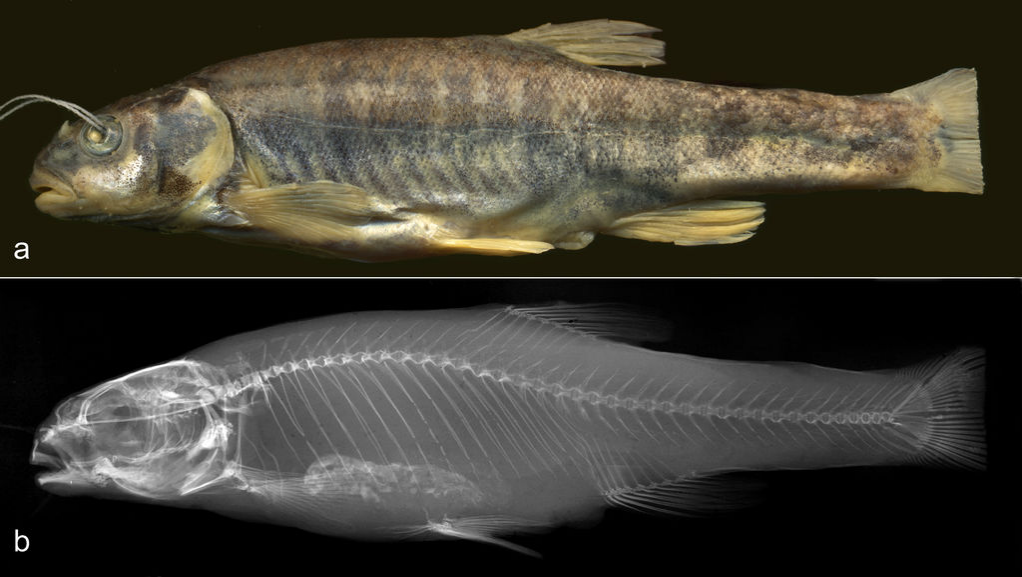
Photo (a) and x-ray (b) of the neotype *Phoxinus
